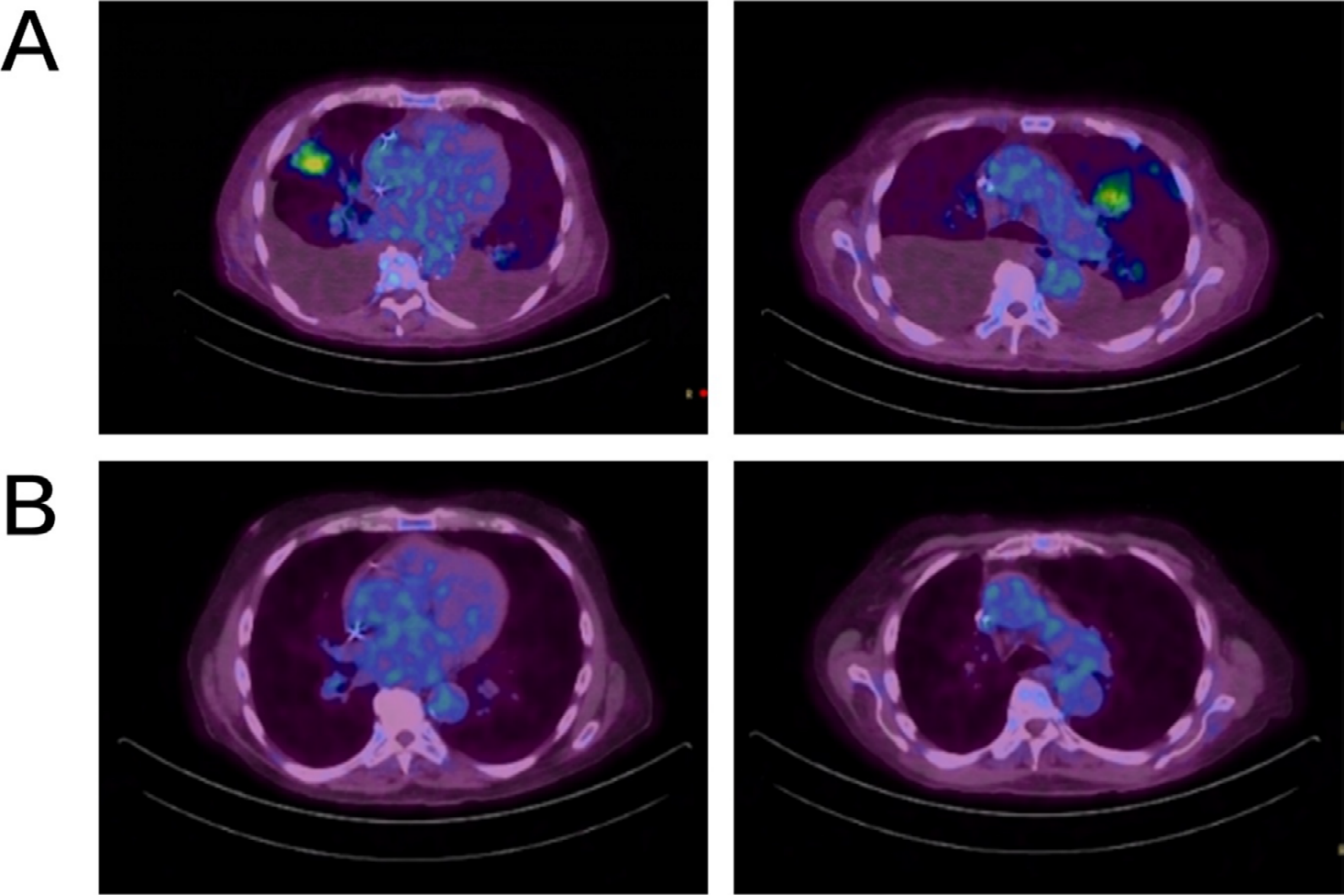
Radiographic response to Olaparib on PET‐CT scans. (A) PET‐CT in October 2021 prior to olaparib therapy showed multiple new hypermetabolic ill‐defined bilateral upper lobe opacities, including a 3.0 × 2.9 cm lobular left upper lobe lung mass (SUV max 5) and a 3.9 × 2.6 cm lobulated right upper lobe lung mass (SUV max 6). (B) Follow up PET‐CT in December 2023 after olaparib therapy demonstrated resolution of the previously noted pulmonary lesion.
